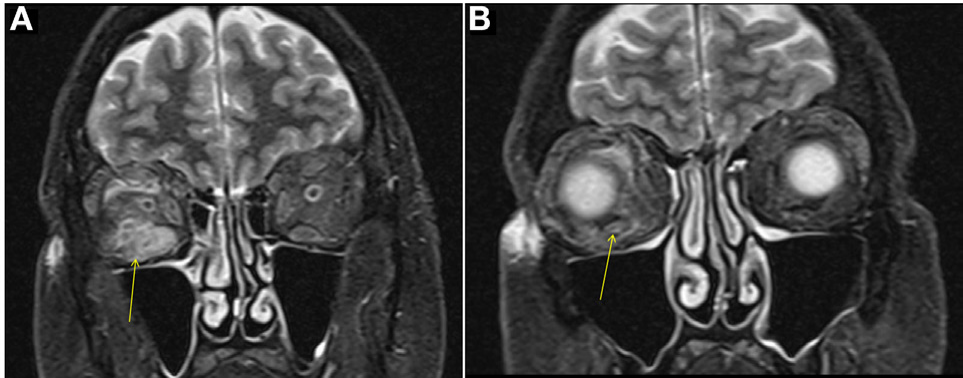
Case 3. Coronal T2W MRI images showing enlargement of the right inferior rectus muscle [arrow in (A)] with surrounding fat stranding, features similar to thyroid-associated orbitopathy (TAO). Focal sparing of the anterior tendinous insertion [arrow in (B)] is another common finding of TAO.
